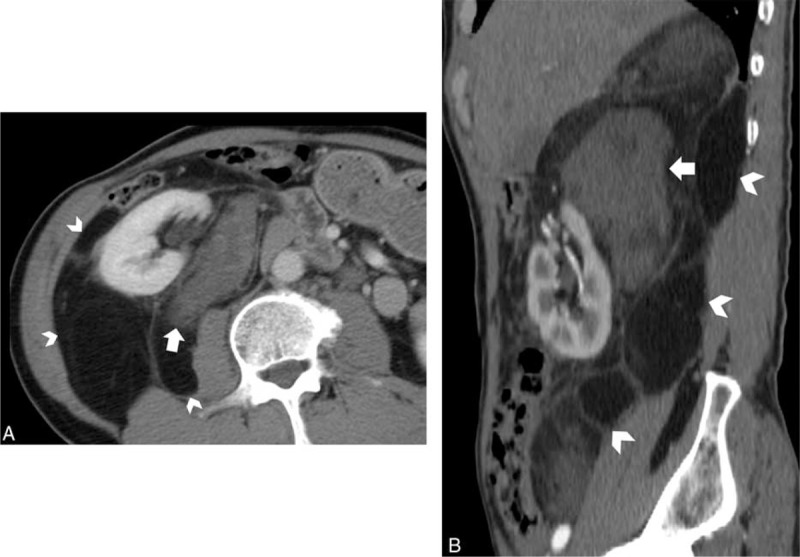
A 57-year-old man with retroperitoneal liposarcoma. A, Axial and B, oblique sagittal contrast-enhanced computed tomography images reveal a large fat-containing mass engulfing the right kidney (white arrowheads) and also a well-defined soft tissue intratumoral nodule with mild enhancement within the tumor (white arrow).
